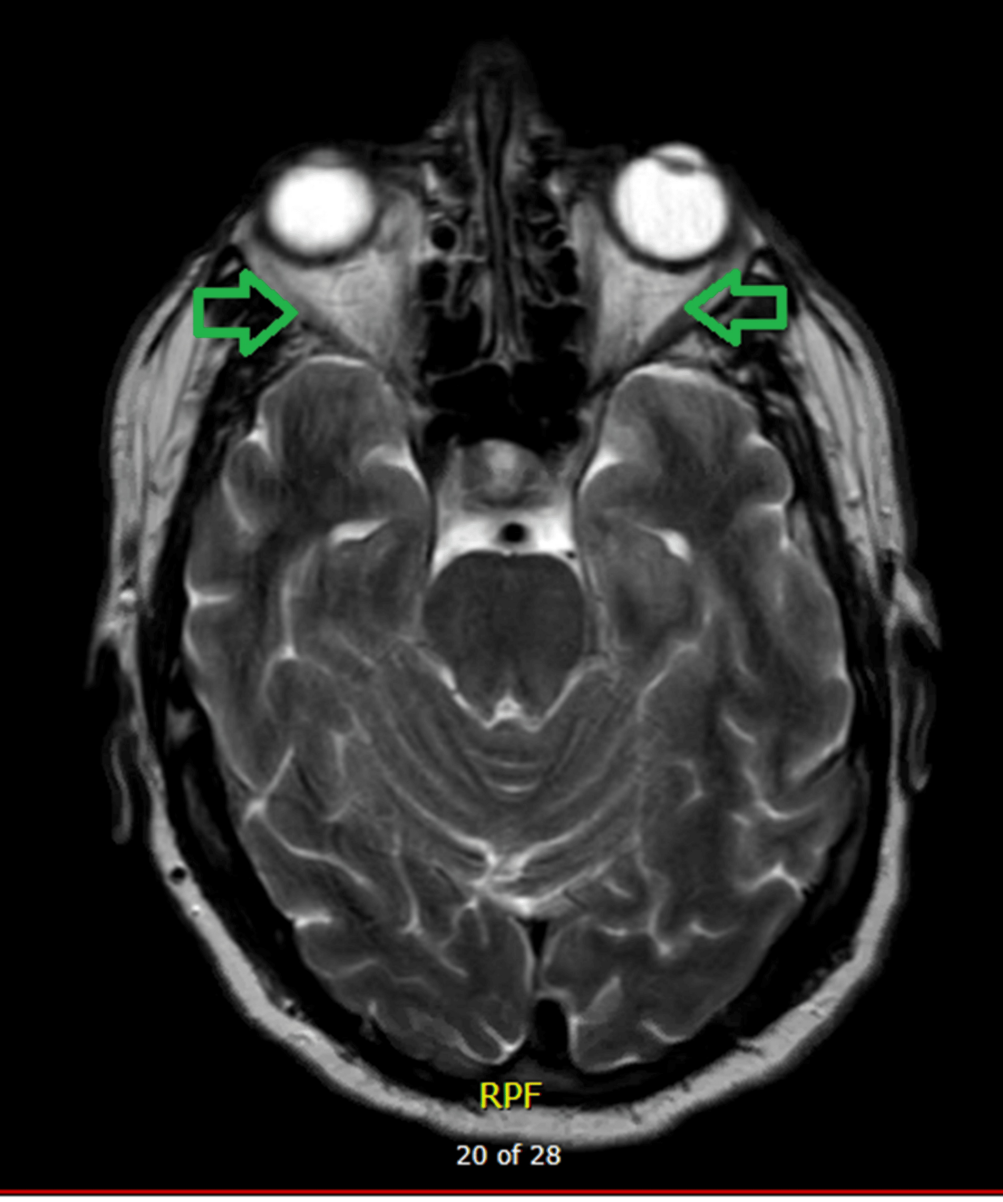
A normal MRI scan of the brain showing no abnormalities within the optic nerves bilaterally (green arrows).
